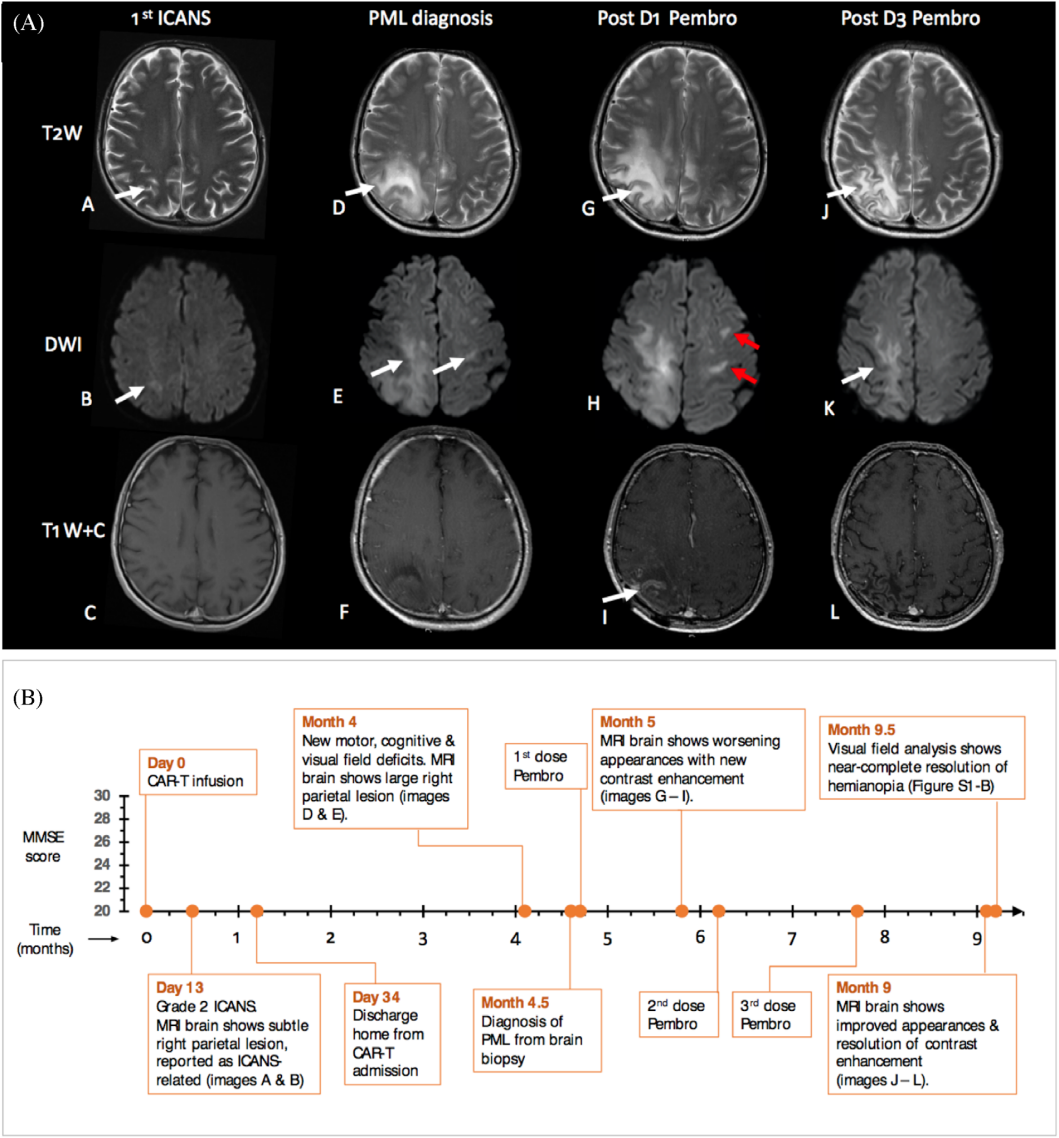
(A) Magnetic resonance imaging of the brain. Axial, gadolinium‐enhanced images showing T2‐weighted (T2W), diffusion‐weighted (DWI) and contrast‐enhanced (T1W+C) sections at 1st episode of immune effector cell‐mediated neurological syndrome (ICANS; A–C), progressive multifocal leukoencephalopathy (PML) diagnosis (D–F), 1 month following 1st pembrolizumab dose (D1; G–I), and after 3rd dose (D3) of pembrolizumab (J–L), which was 5 months after 1st infusion. Panels A and B show a small focus of T2‐weighted signal abnormality in the right deep parietal white matter, demonstrating mild restriction of diffusion on DWI (B) at ICANS diagnosis. After the first pembrolizumab dose, note the relative increase in the size of PML lesions (G) with the progression of restricted diffusion (H) including new contralateral areas (red arrows) and new contrast enhancement (I), which had improved (J, K) or resolved (L) on interval imaging a month after D3. (B) Timeline of clinical and radiological events post CAR‐T infusion (x‐axis) with serial mini‐mental state examination (MMSE) scores (y‐axis). At presentation, MMSE score was reduced (25/30), declined further after 2 weeks (22/30), but improved over 4 months (to 29/30) following pembrolizumab infusion. Image references refer to (A)
